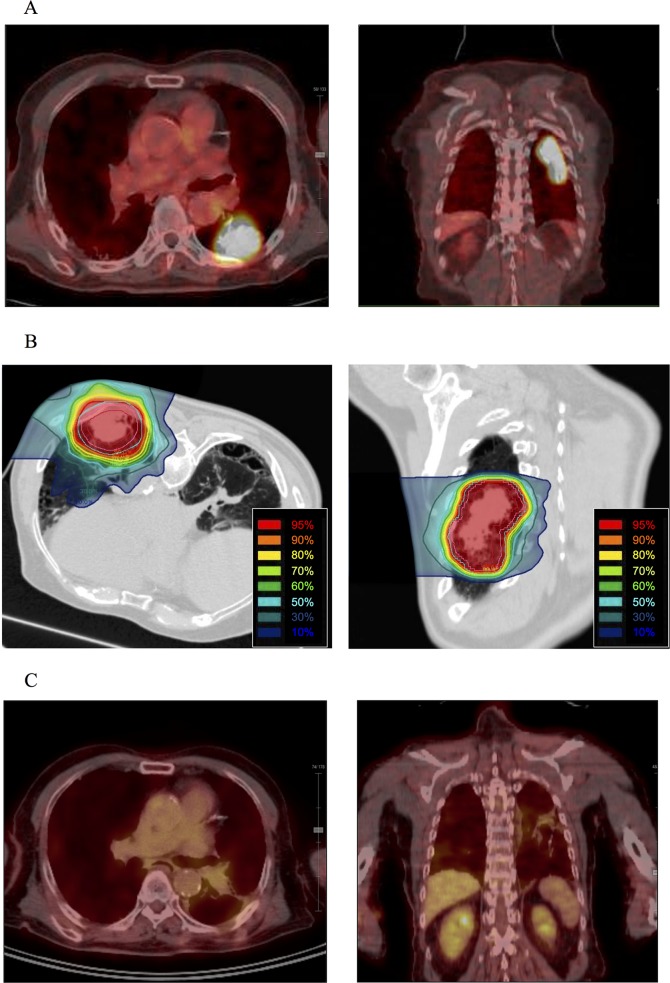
A representative case treated with carbon-ion radiotherapy. (A) A 91-year-old man with T3N0M0 lung squamous cell carcinoma. A rapidly growing tumor was 73 mm in diameter. (18) F-Fluorodeoxyglucose positron emission tomography (FDG-PET) images revealed that the tumoral maximum standardized uptake value was 12.1 and tumor to normal tissue (T/N) ratio was 3.8. The patient was considered inoperable because of his age and chronic obstructive pulmonary disease. (B) Carbon-ion radiotherapy was performed using a respiratory-gated technique with 60 Gy (relative biological effectiveness) in 4 fractions. The GTV, CTV, and PTV are represented by the red, cyan, and magenta lines, respectively. (C) Following treatment, asymptomatic grade 1 radiation pneumonitis developed but additional treatment was not required. Two years after carbon-ion radiotherapy, FDG-PET images demonstrated reductions in the maximum standardized uptake value from 12.1 to 2.5 and in the T/N ratio from 3.8 to 1.0. Distant or lymph node metastases were not detected. The patient was still alive without disease progression at 3 years after carbon-ion radiotherapy.
